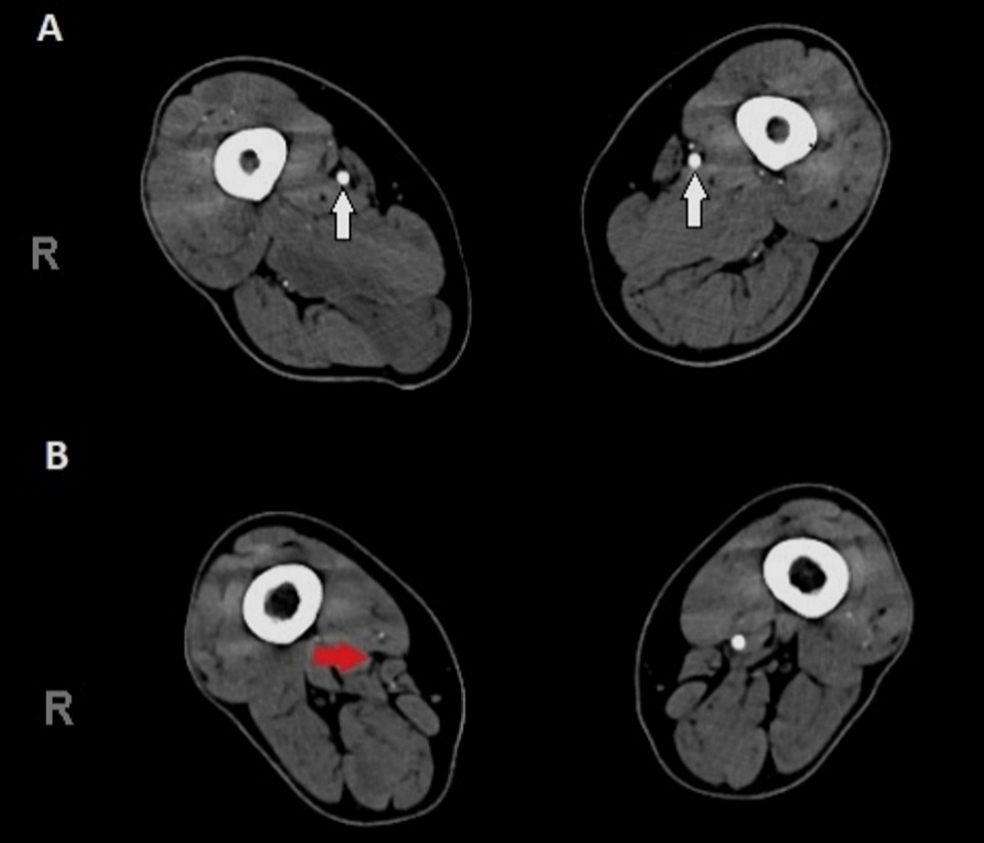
CT Angiography of lower limbs 2A, CT section showing normal contrast refilling at the level of the superficial femoral artery of both lower limbs (white arrows) 2B, Complete occlusion of the right lower third of superficial femoral artery and down arteries (red arrow)
